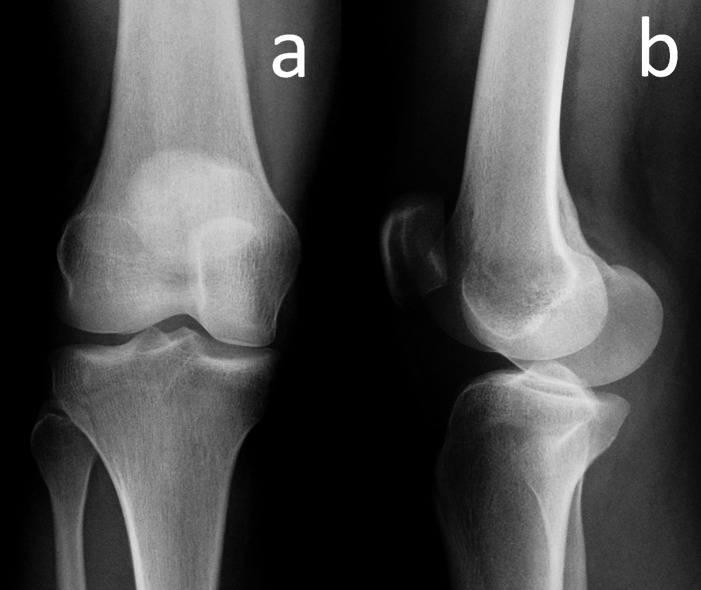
Anteroposterior and lateral knee radiographs
